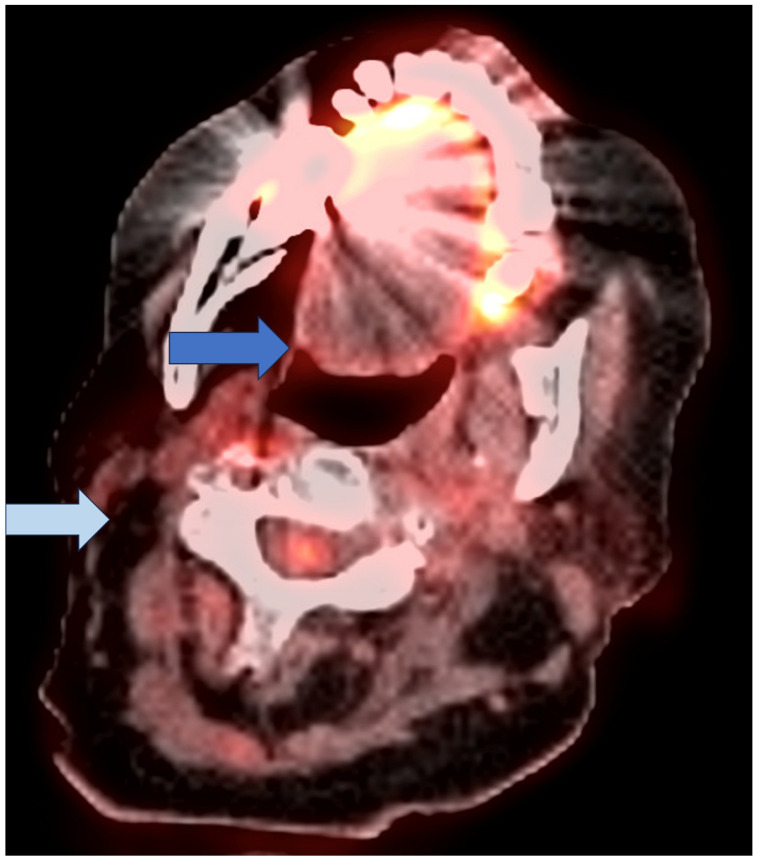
Axial fused image of a post-therapy FDG PET/CT in a 74-year-old woman status post-radiation of right tonsil cancer which demonstrates no residual FDG uptake in the right tonsil (dark blue arrow) nor in the previously avid nodes (all sites below mediastinal blood pool, marked by light blue arrow). This case represents a complete metabolic response by all criteria. More specifically, this exam would be assigned CR by PERCIST as the tumor has disappeared; H1 (negative) by Hopkins as the uptake is less than the IJV; N1 (no evidence of recurrence) by NIRADS as there is no uptake of visible mass on CT; M1 (negative) by MDS as the uptake is less than the mediastinal blood pool; C1 (negative) by Cuneo for the same reason; and P1 (negative) by Porceddu as there is no residual uptake above the mediastinal blood pool in the nodes.
